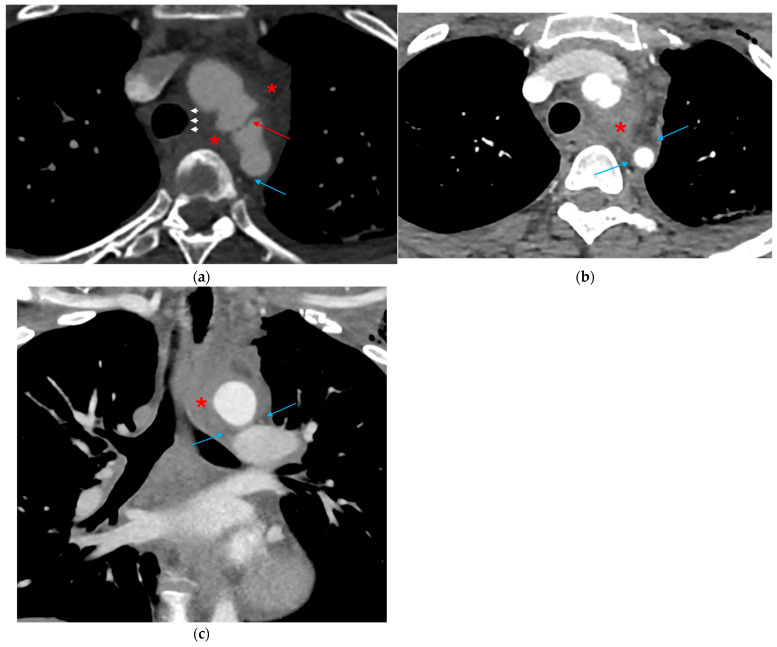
Traumatic aortic injury with periaortic hematoma. (a,b) Axial and (c) coronal CTA images show an intimal traumatic defect (red arrow) with associated periaortic fat stranding (blue arrows), periaortic hematoma extending across the superior, medium and posterior mediastinum (red asterisks), and right deviation of trachea (white arrowheads).
